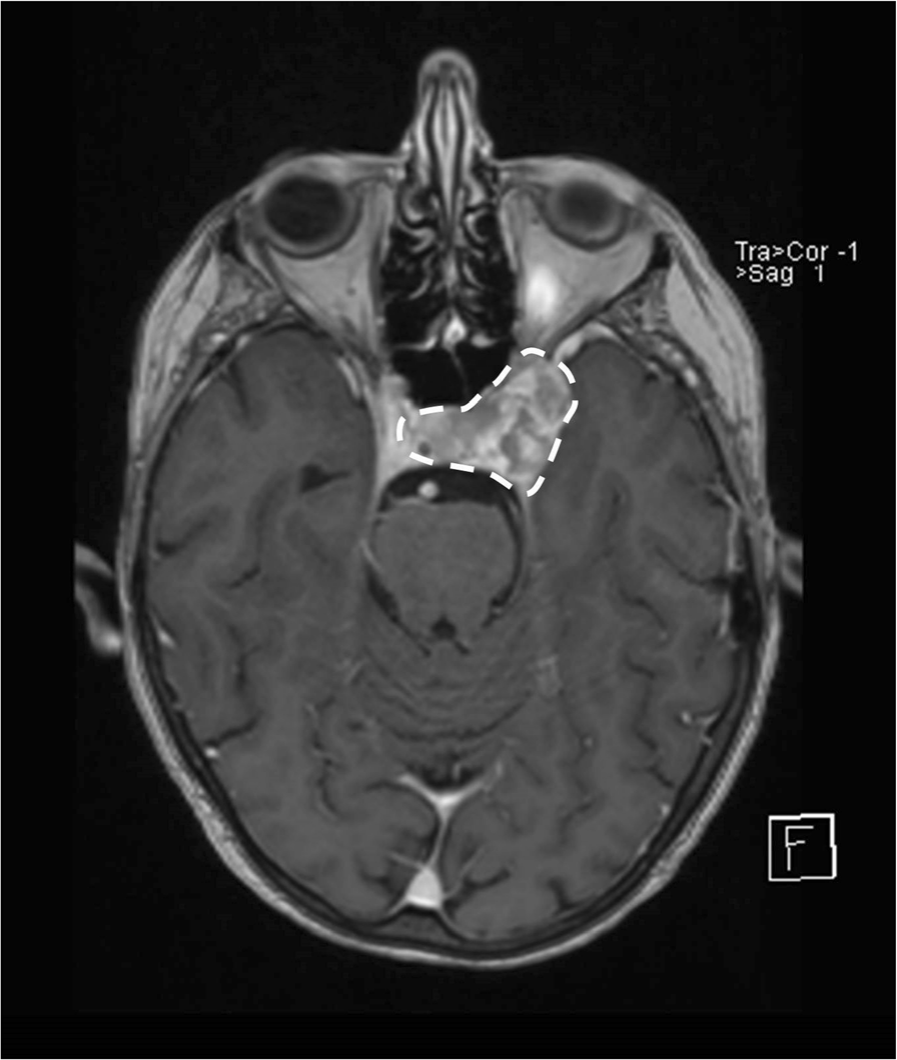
Magnetic resonance imaging of the head with contrast demonstrating an infiltrative enhancing mass in patient’s left skull base extending into cavernous sinus measuring 2.6 × 1.8 cm (encompassed within *dotted white line*). Medially, this is inseparable from the pituitary gland
